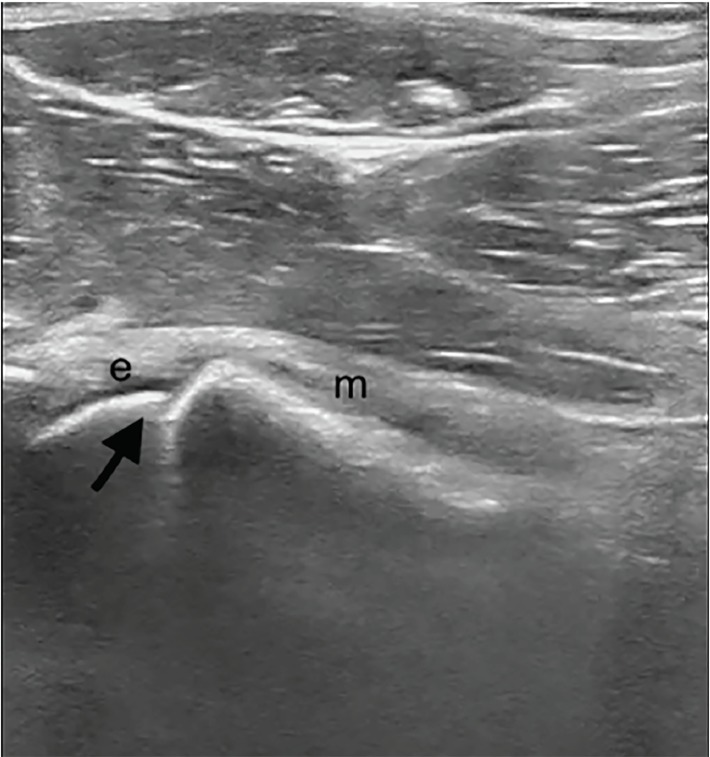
Point-of-care ultrasound image of left hip showing displacement of epiphysis(e) from metaphysis (m) – the physeal step (arrow).
